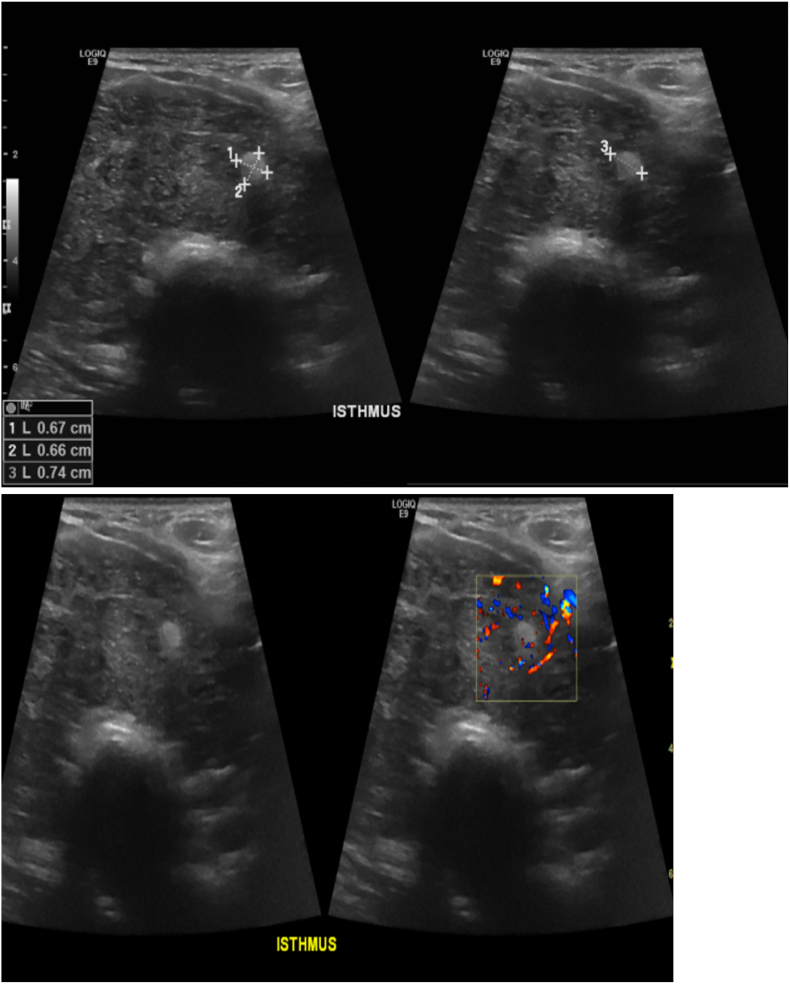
Thyroid ultrasound showing hyperechoic nodule measuring 0.74 cm in maximum dimension.
